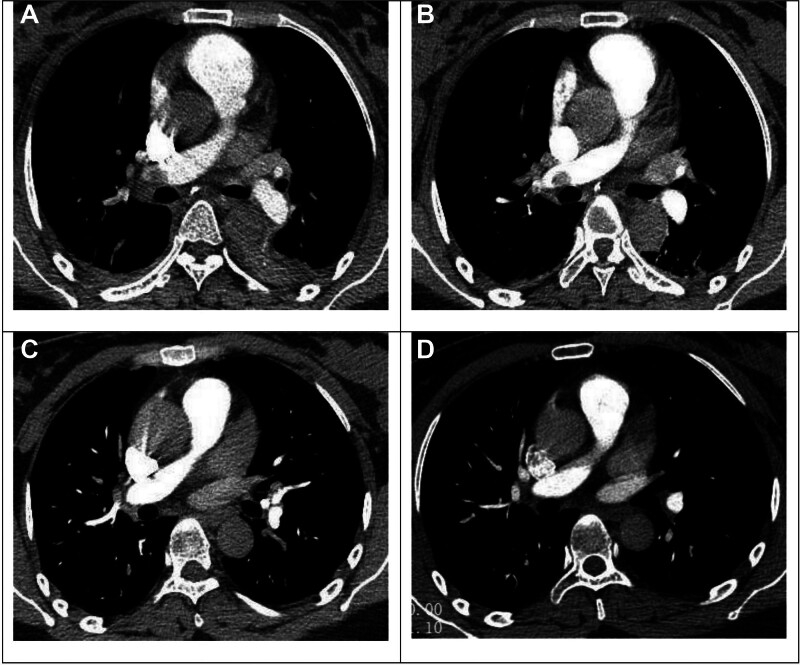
CTPA imaging presentation. (A) A shadow of a filling defect was seen in the lumen of the main trunk of the right lower pulmonary artery and the primary and secondary major branches, and thromboembolic formation was considered. (B) Thromboembolism formation in the main trunk of the right lower pulmonary artery and the lumen of the primary and secondary major branches, which was better than Figure A. (C) A little thromboembolism in the lumen of the primary and secondary major branches of the right lower pulmonary artery, significantly better than Figure B. (D) CTPA showed no obvious abnormality. CTPA = computed tomography pulmonary angiography.
